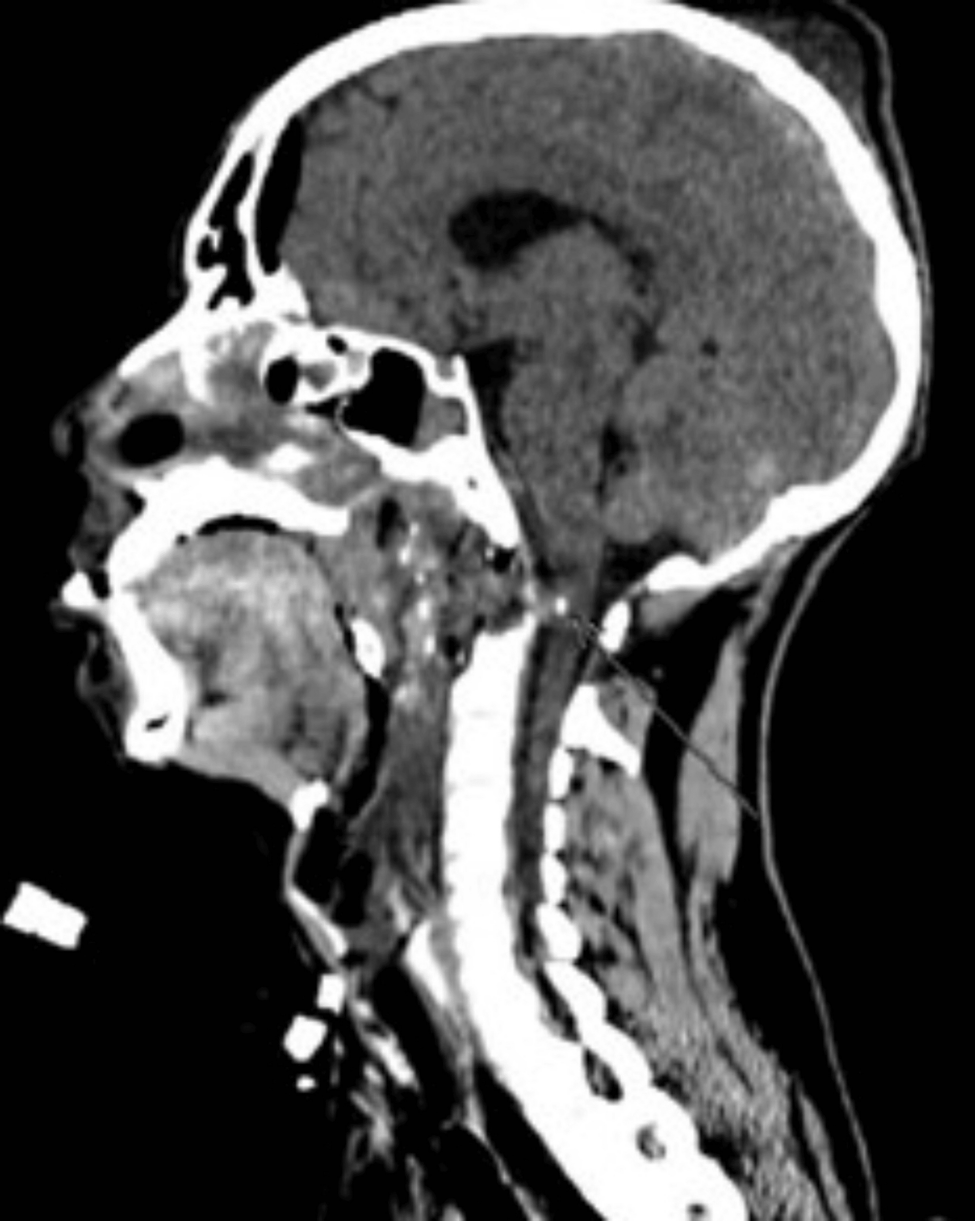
The postoperative sagittal CT scan confirms the absence of the metallic foreign body (bullet), with no residual fragments observed along its trajectory. The odontoid process (C2) has been partially resected. CT, computed tomography
